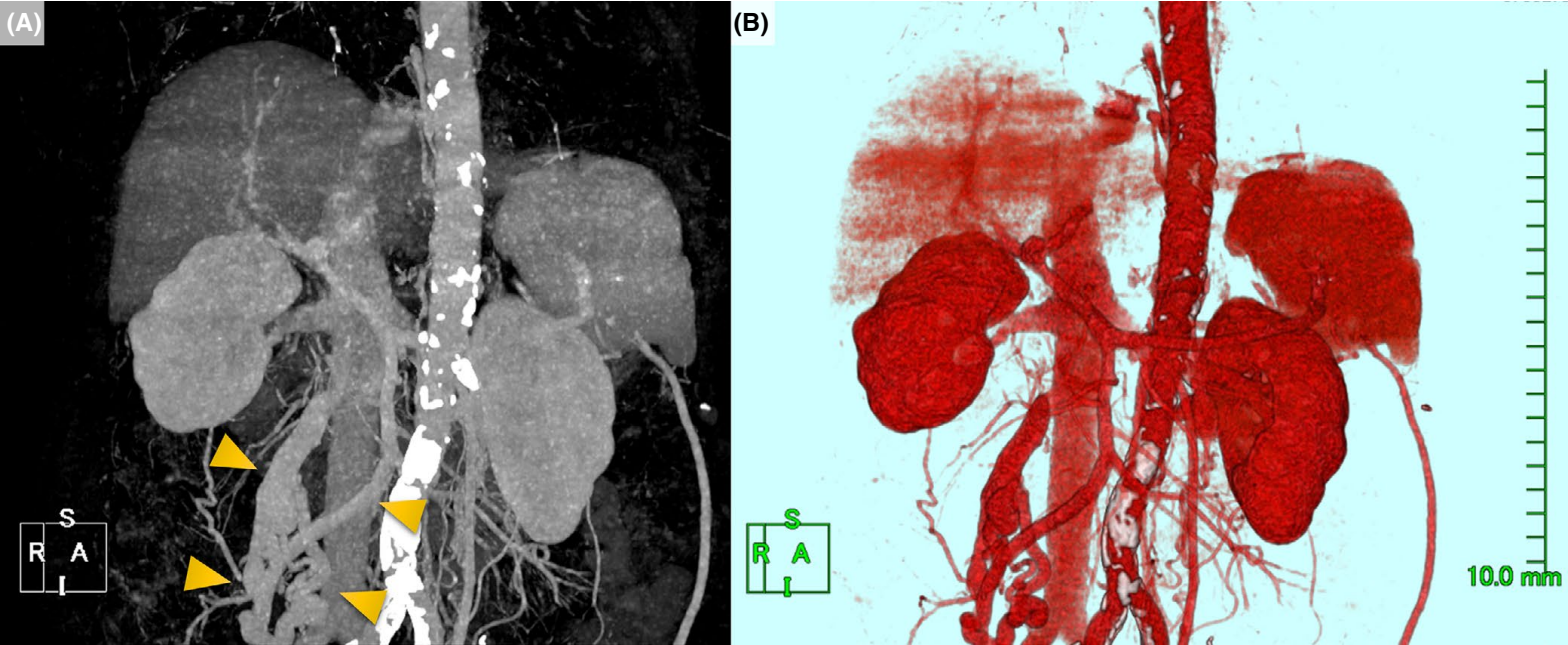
(A, B) 3D‐reconstructed computed tomography images revealing extrahepatic portosystemic shunt connecting superior mesenteric veins with inferior vena cava (arrowheads). Figure 1B depicts the vasculature only
